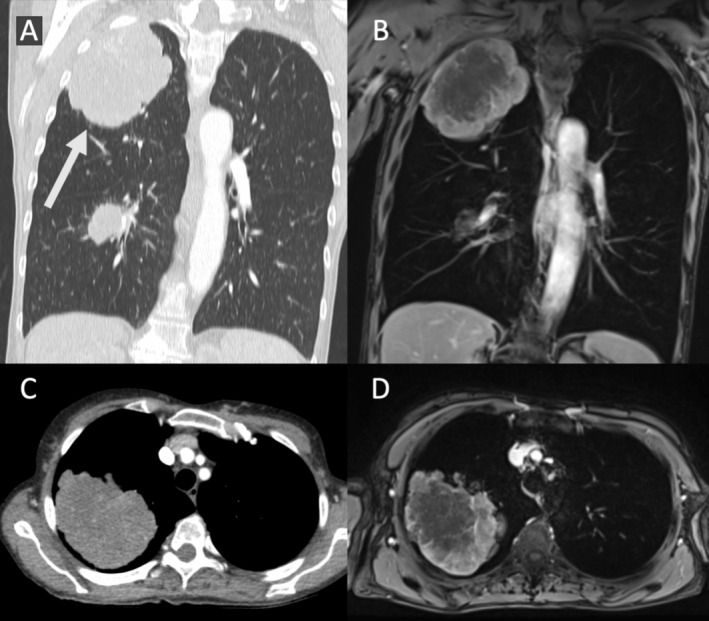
A 63‐year‐old female patient with a mass (arrow) in the right upper lung lobe and suspected lung cancer. Transverse and coronal contrast‐enhanced CT (A and C) was used to plan a CT‐guided biopsy, which produced only necrotic material. A second biopsy after contrast‐enhanced MRI (B and D: contrast‐enhanced 3D‐gradient echo fat‐suppressed T1‐weighted images) allowed for targeted biopsy of vital tissue and revealed a renal cell carcinoma metastasis.
